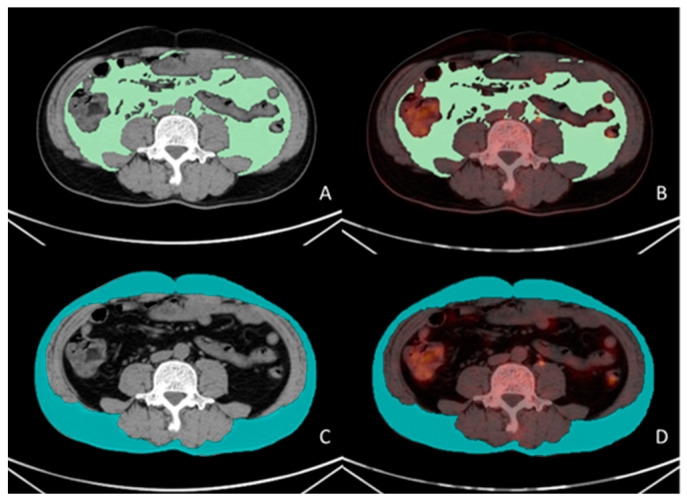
Measurement methodology for VAT and SAT SUVmean. A 56-year-old male patient with gastric cancer underwent preoperative ^18^F-FDG PET/CT examination. Visualized using LIFEx software (version 7.4.0). VAT (green color in (A)) and SAT (blue color in (C)) regions were delineated on three consecutive CT slices at the L4–L5 vertebral level based on the CT attenuation range (−50 to −150 Hounsfield units [HU]). The CT-defined VAT and SAT regions were subsequently co-registered with corresponding PET/CT fusion images (green color in (B), blue color in (D)), yielding a VAT SUVmean of 0.43 and an SAT SUVmean of 0.32.
